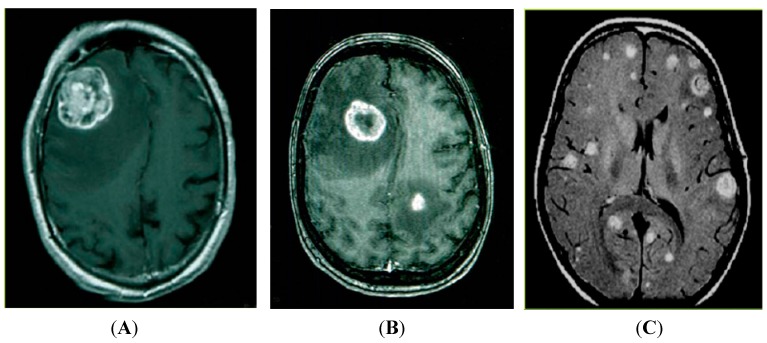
Magnetic resonance imaging (MRI) detection of brain metastasis. (A) Solitary lesion; (B) Oligometastasis; (C) Multiple brain metastases.
